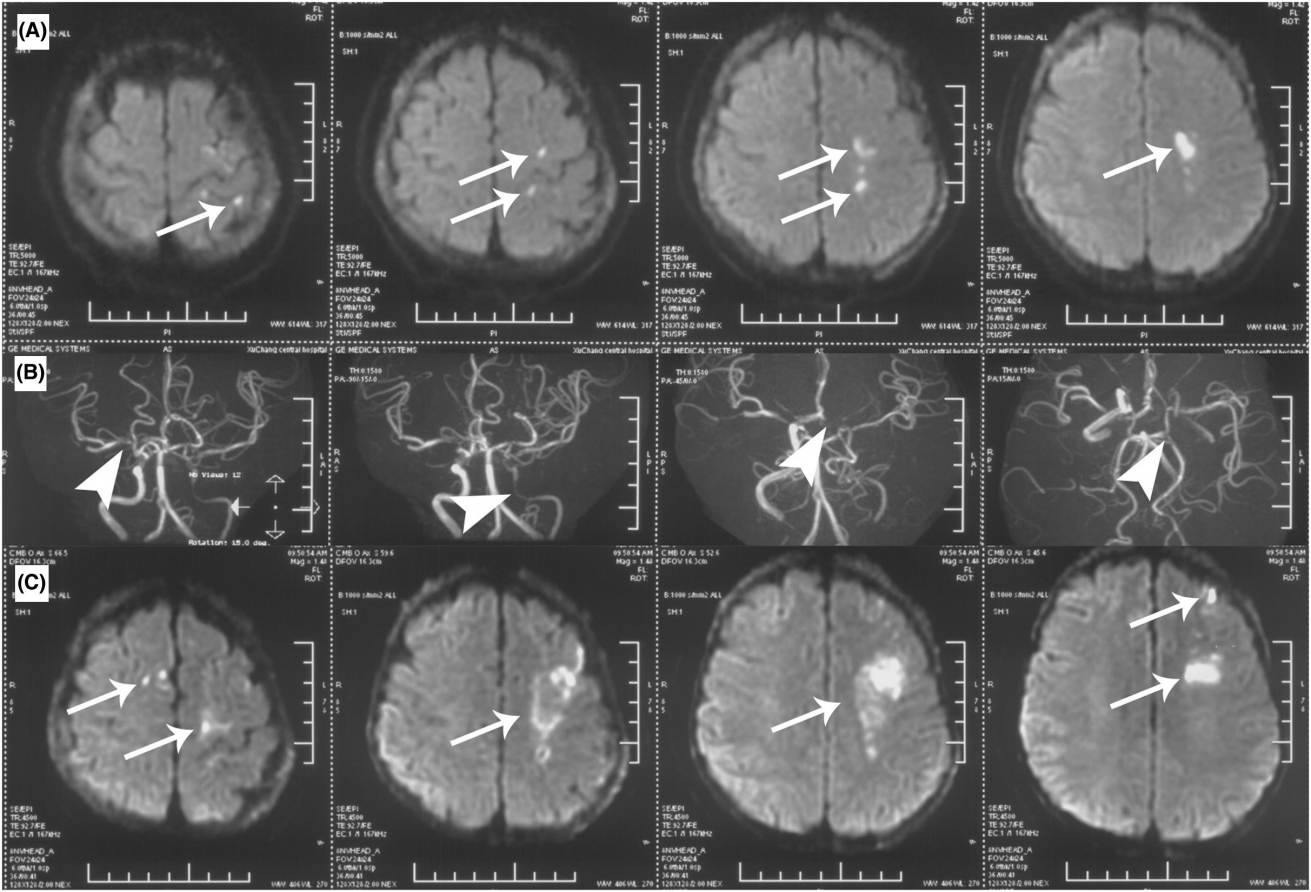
(A) Initially axial DWI of brain showed acute infarction in left frontoparietal lobe (long white arrow). (B) MRA demonstrated vascular stenosis in the proximal part of bilateral MCA, moderate to severe stenosis in left ICA, A1 segment of left ACA and proximal segment of posterior cerebral artery (PCA) (short white arrow). (C) The second axial brain DWI revealed multiple acute cerebral infarctions in right frontal regions and extended ischemic lesions in left frontoparietal lobe (long white arrow)
